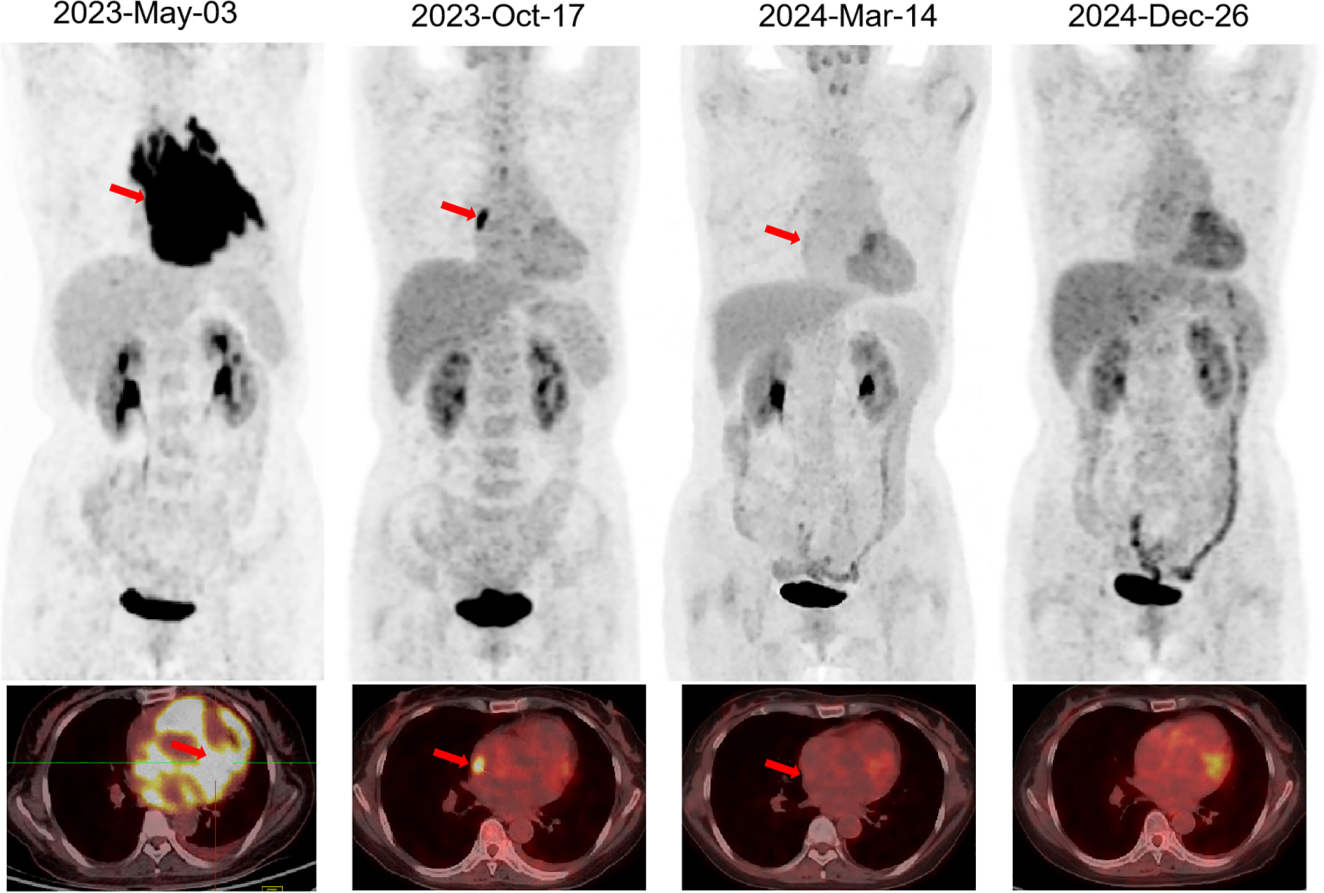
PET-CT evaluation of PCL. The arrow indicates the lesion. PET-CT, Positron Emission Tomography-Computed Tomography; PCL, primary cardiac lymphoma.
